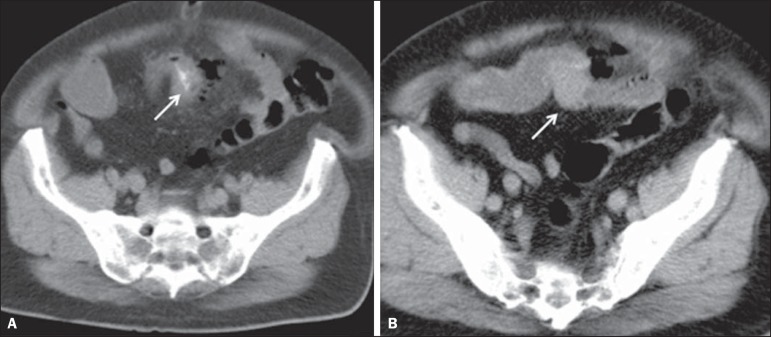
A 74-year-old male patient. A CT scan of the abdomen, showing a foreign
